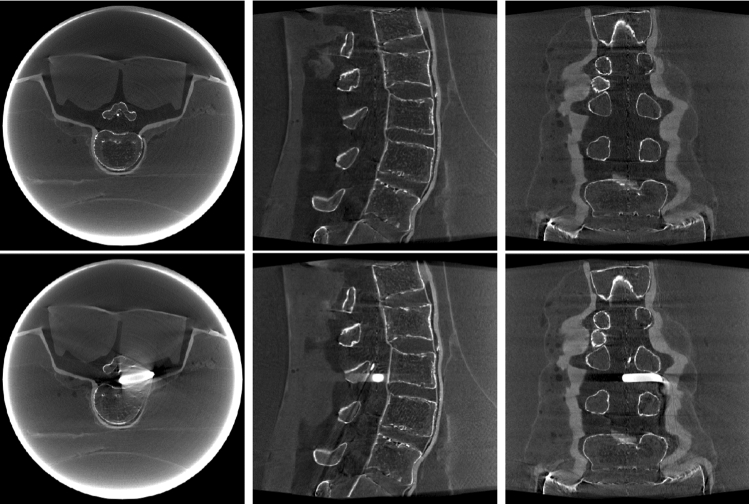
Upper Row: 1st CBCT used as prior. Bottom Row: 2nd CBCT, projections used in calibration
